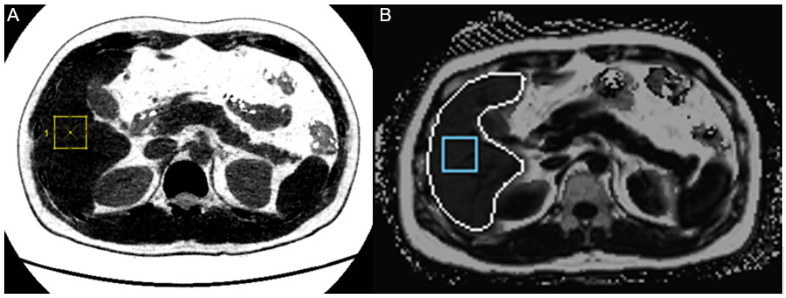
An example of fat quantification using (A) an FVF map and (B) MRI-PDFF in a 33-year-old man with fatty liver (MRI-PDFF = 6.5%). The FVF map yielded a value of 5.2%.
